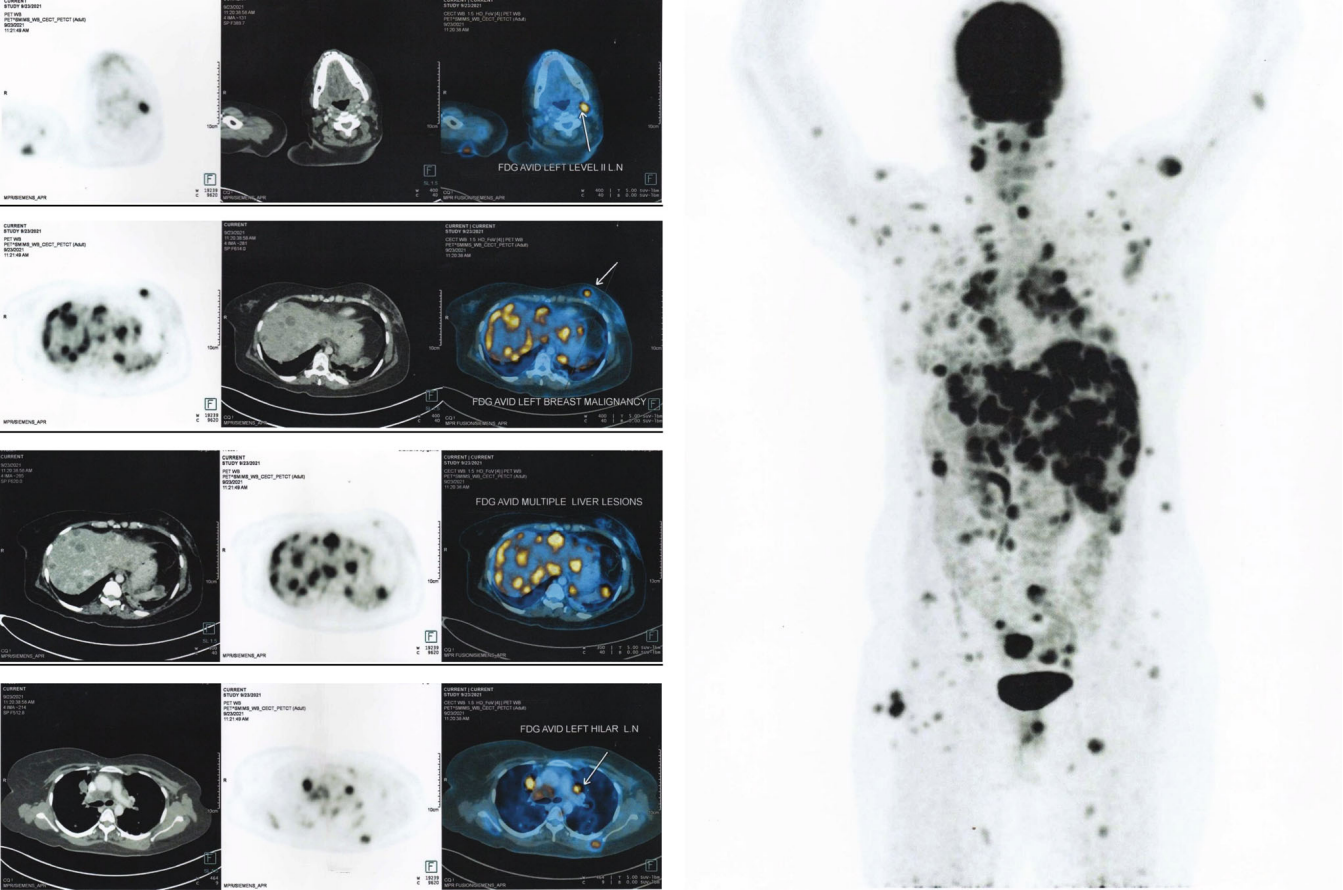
PET scan images showing extensive FDG avid metastases all over the body.
